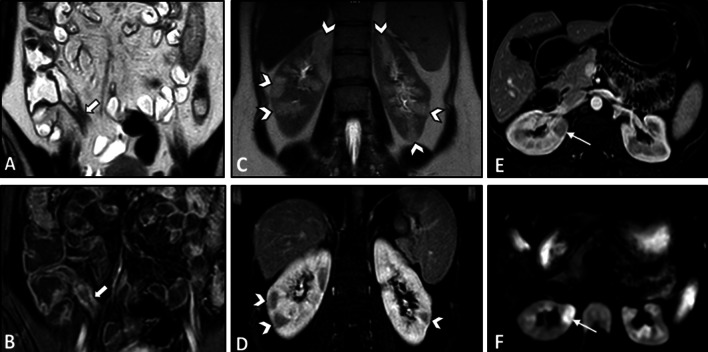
Intestinal vasculitis in a 39-year-old female presenting with episodic abdominal pain and findings of non-specific terminal ileitis in ileocolonoscopy examination. MRE was obtained. Coronal T2-W and post-contrast T1-W images (A, B) display mild mural thickening and hyperenhancement at distal terminal ileum (thick white arrows). Coronal T2-W and post-contrast T1-W images (C, D) reveal multiple peripheral wedge-shaped hyperintense areas at both kidneys, showing decreased enhancement on delayed phase (white arrowheads). Axial post-contrast T1-W and corresponding DWI images (E, F) show a hypoenhancing lesion at the right renal cortex with diffusion restriction (thin white arrows). Vasculitis was suspected as the underlying cause. Afterward, positive results for antineutrophil cytoplasmic antibody (ANCA) and inflammatory markers were consistent with the diagnosis of ANCA-associated vasculitis
